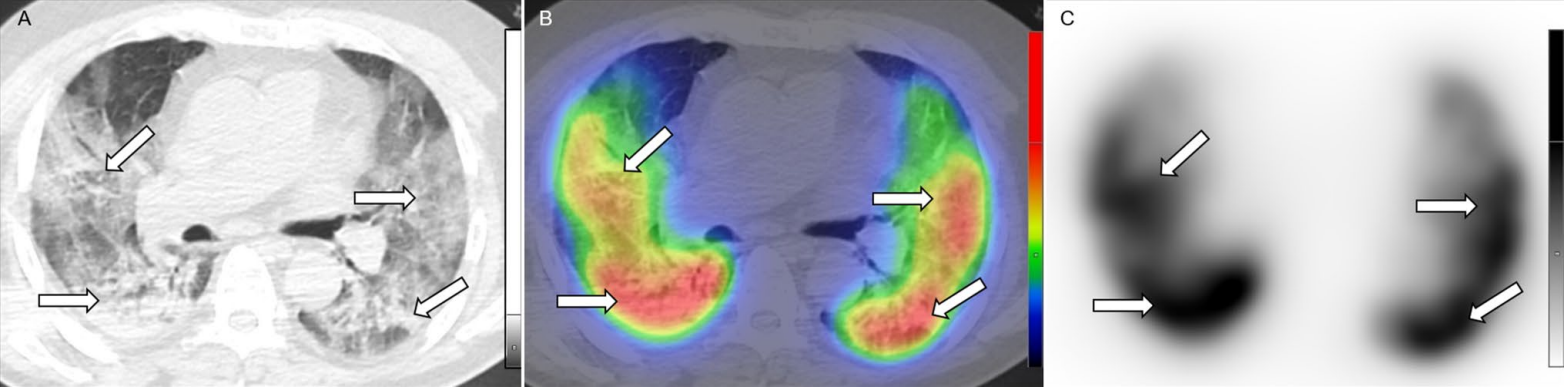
Axial low-dose CT (a), fused Q-SPECT/CT (b), and SPECT scans (c) of a COVID-19 patient. Fused SPECT/CT scan reveals remarkably extensive hyperperfused opacities in both lungs (shown with *white arrows*) compared to the relatively preserved lung areas. *CT* computed tomography, *Q-SPECT/CT* perfusion single-photon emission computed tomography/computed tomography, *COVID-19* coronavirus disease 2019
